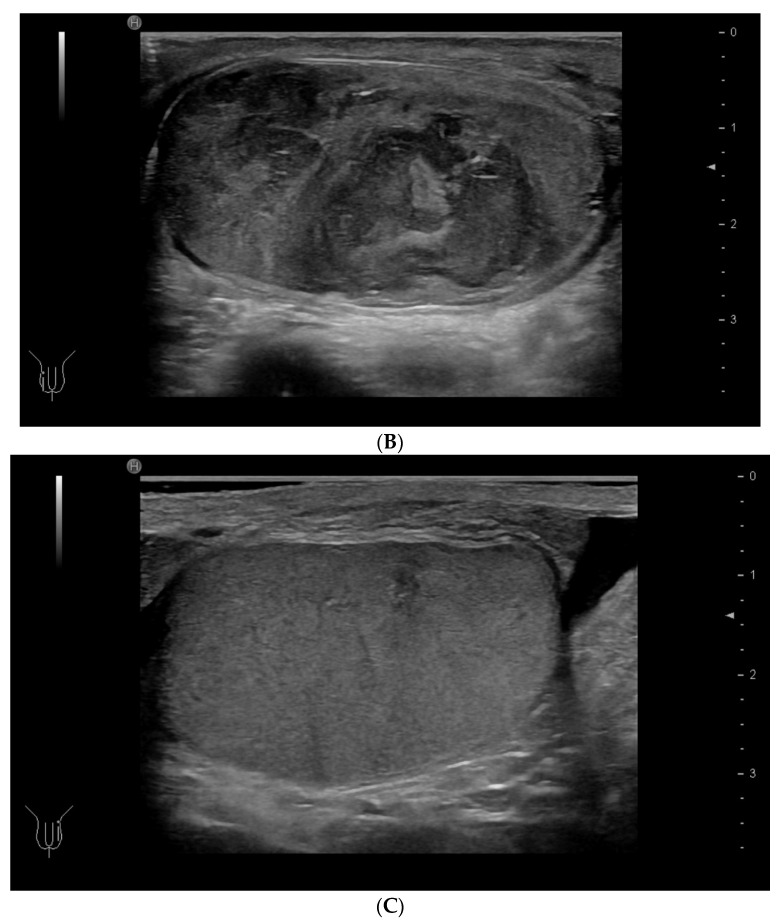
Ultrasound images of a 39-year-old male. The indication for ultrasound investigation was suspected orchitis or malignancy. The patient experienced pain in the right testicle for more than 2 days. The patient was seen in the emergency room for assessment of epididymitis and started treatment with ciprofloxacin. There was no improvement with treatment, and the right testicle became more tender and swollen. The ultrasound showed no signs of epididymitis (A). The right testicle (left side of the figure shows the elastogram, and B-mode is seen on the right side). The testicle revealed a right-sided tumour, which appeared highly suspicious using elastography (B). The B-mode image, showing a tumour (C). B-mode image of the left testicle, showing normal tissue (The pathology showed a seminoma testicular tumour with 2% of choriocarcinoma. The patient underwent surgery for removal of the right testis.
